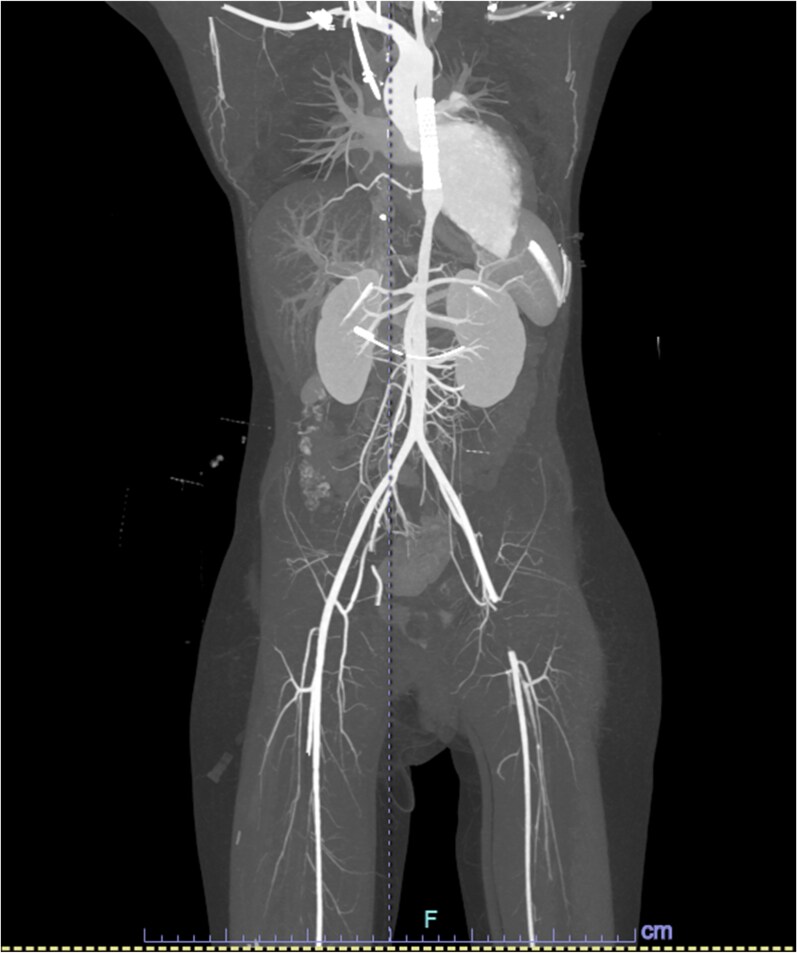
The reconstructed computed tomography angiogram images (pre-aspiration mechanical thrombectomy) showing thrombosis of left common femoral artery, with visible aortic stents after coarctation of aorta stenting.
